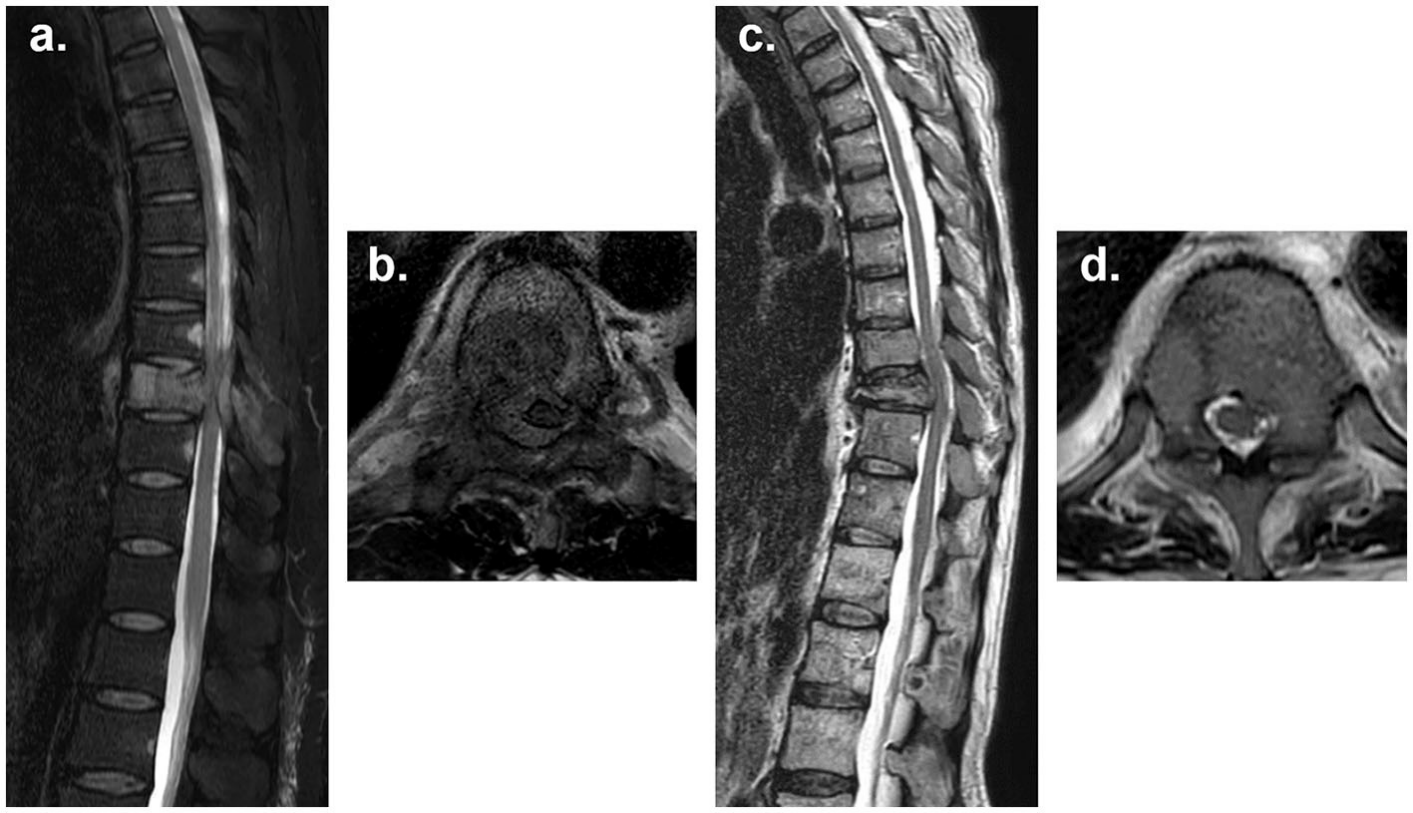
(a, b) T2-weighted MR images demonstrate a spine metastasis in clinical scenario 1. Scenario 1 describes a 55-year-old male patient with hepatocellular carcinoma who presented with a week-long history of leg weakness due to spinal cord compression (Bilsky grade 3) at the T7 level. The patient had a single spinal metastasis, a SINS score of 9, a KPS score of 70, an expected survival time of <6 months, and no further plan for systemic treatment. (c, d) T2-weighted MR images demonstrate spine metastases in clinical scenario 2. Scenario 2 describes a 60-year-old female patient with breast cancer who presented with severe back pain due to a pathologic compression fracture at the T10 level with spinal cord abutment (Bilsky grade 1c). The patient had multiple spinal metastases, a SINS score of 9, a KPS score of 90, an expected survival time of more than 6 months, and a plan for further systemic treatment.
